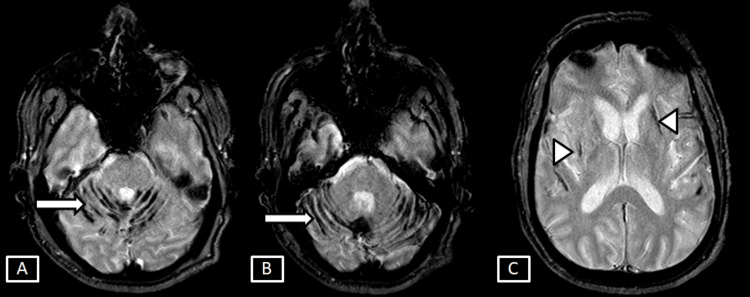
Case 3 MRI brain T2 GRE sequences. Images show symmetrical linear areas of blooming along the bilateral cerebellar foliae and vermis (A, B) represented by the white arrows. Also,  hemosiderin deposition is seen along  the chronic infarct changes of the right lentiform nucleus and left external capsule represented by the white arrowheads. GRE: Gradient Echo
